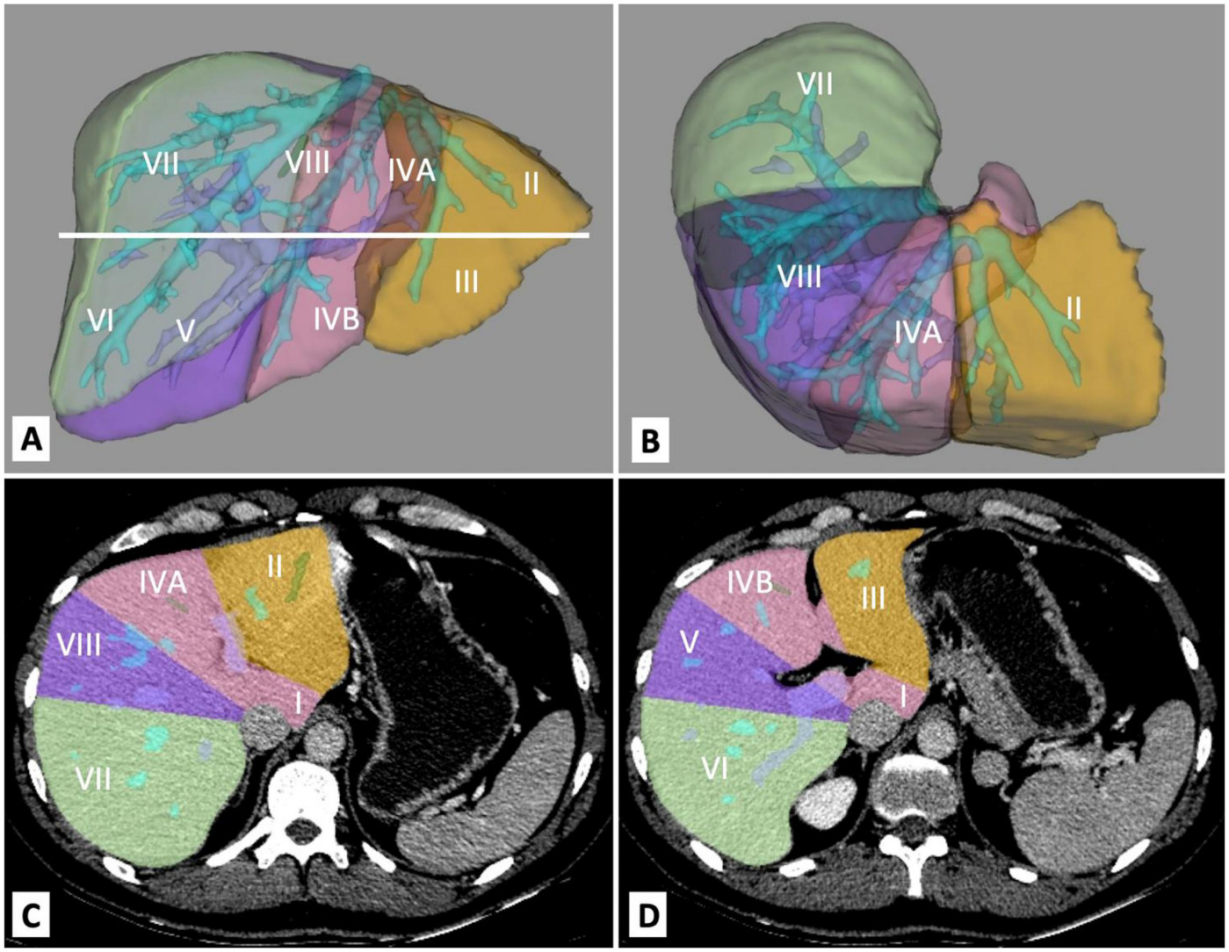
3D computed tomography reconstruction of the liver with segmental anatomy: (A) anterior view of the liver; (B) superior view of the liver. Liver segments in computed tomography: (C) superior cross-section of the liver; (D) inferior cross-section of the liver.
